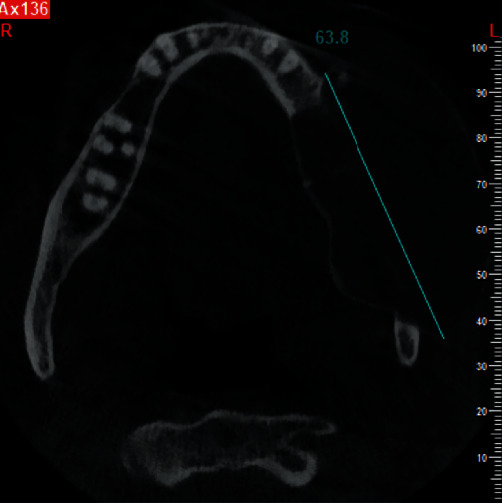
Anteroposterior size of the lesion in axial CBCT section.
